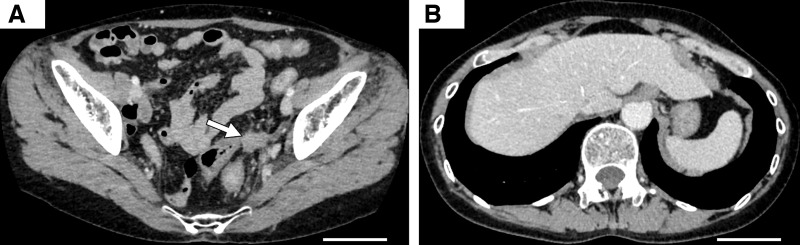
CT after four courses of FOLFOXIRI + bevacizumab (scale bar, 10 cm). (A) Significant reduction of the primary tumor, measuring 22 × 18 mm, with scar formation was observed (arrow). (B) Marked regression of liver metastases was noted.
